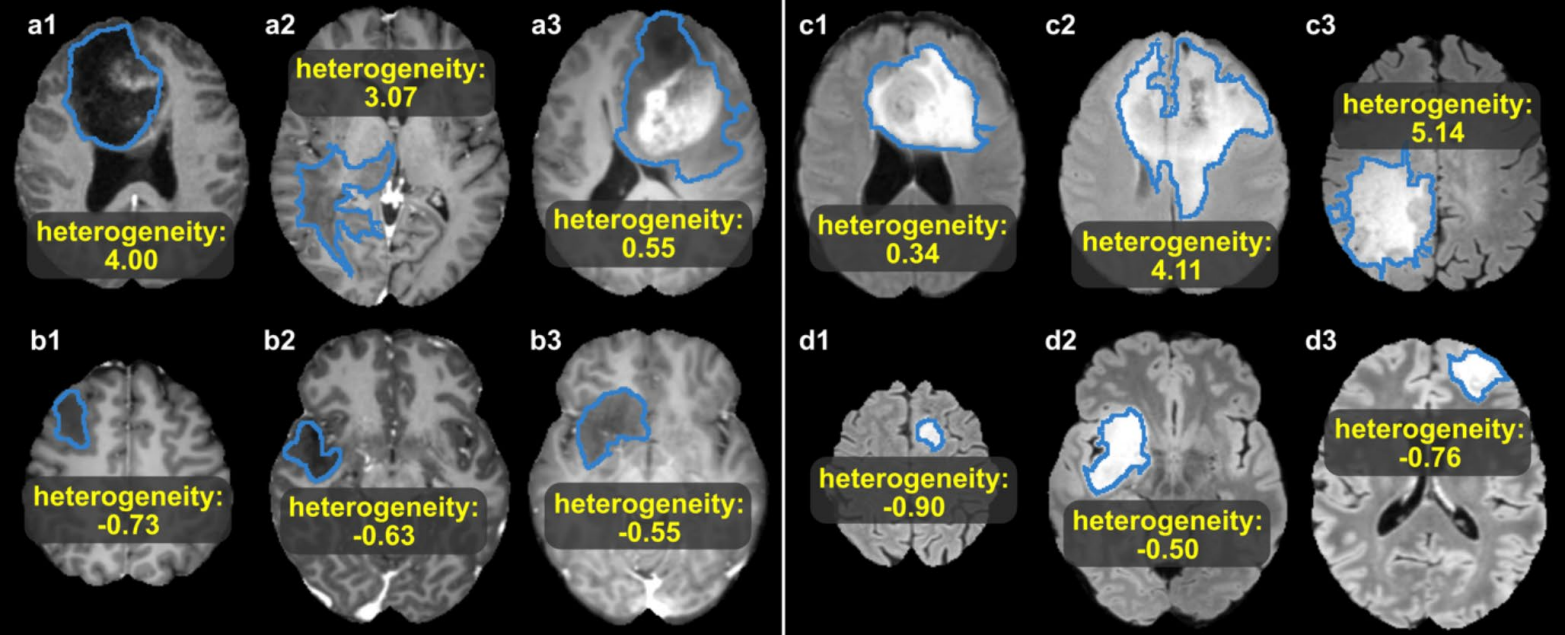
Visualization of 12 case examples of the radiomics tumor heterogeneity feature. (a1-3) visualizes patients with high values of the feature on T1c, (b1-3) patients with low values on T1c. (c1-3) visualizes patients with high values of the feature on FLAIR, (d1-3) patients with low values on FLAIR. Note that segmentations are done in FLAIR/T2-weighted images and may appear larger than the volume depicted in T1c images
